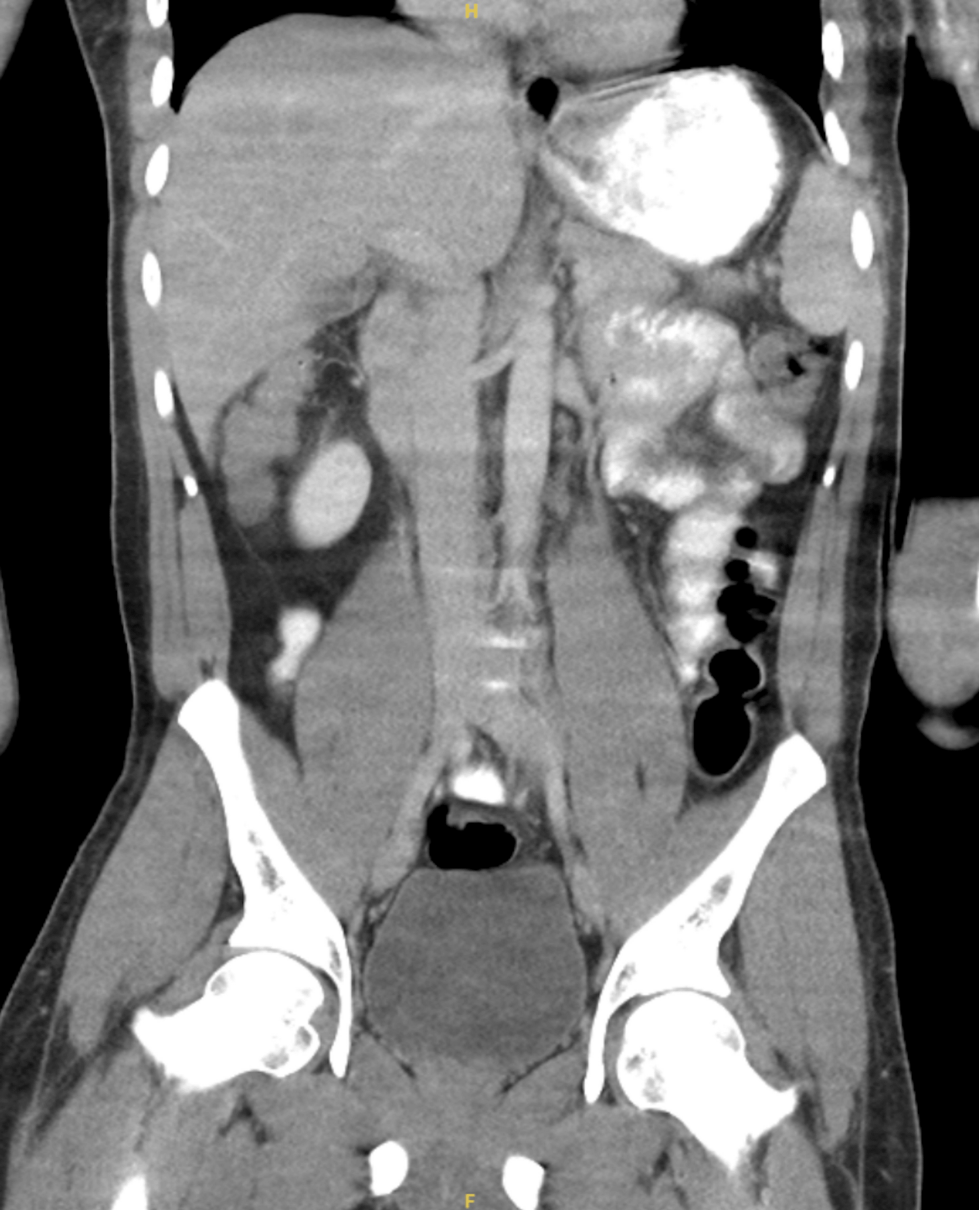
Coronal CT of the abdomen and pelvis demonstrating no acute intra-abdominal pathology, lymphadenopathy, splenomegaly, or mass lesion CT: computed tomography
